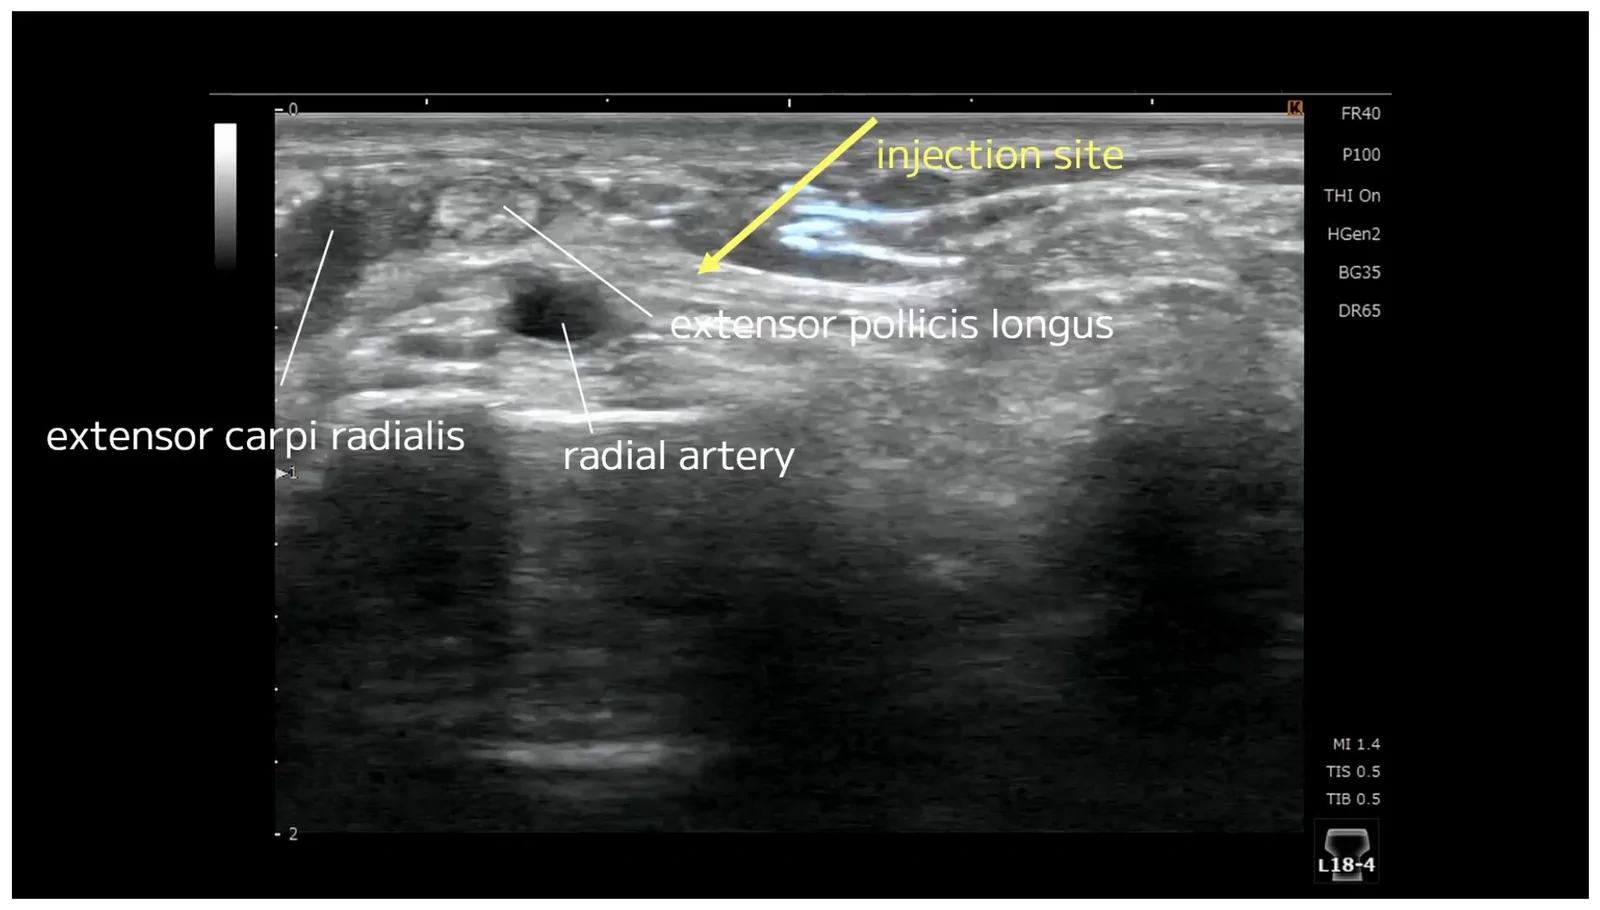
Point 6—radial artery/EPL–ECRL–ECRB crossing (snuffbox): ultrasound-guided fascia hydrorelease; yellow arrow indicates the injection site.
